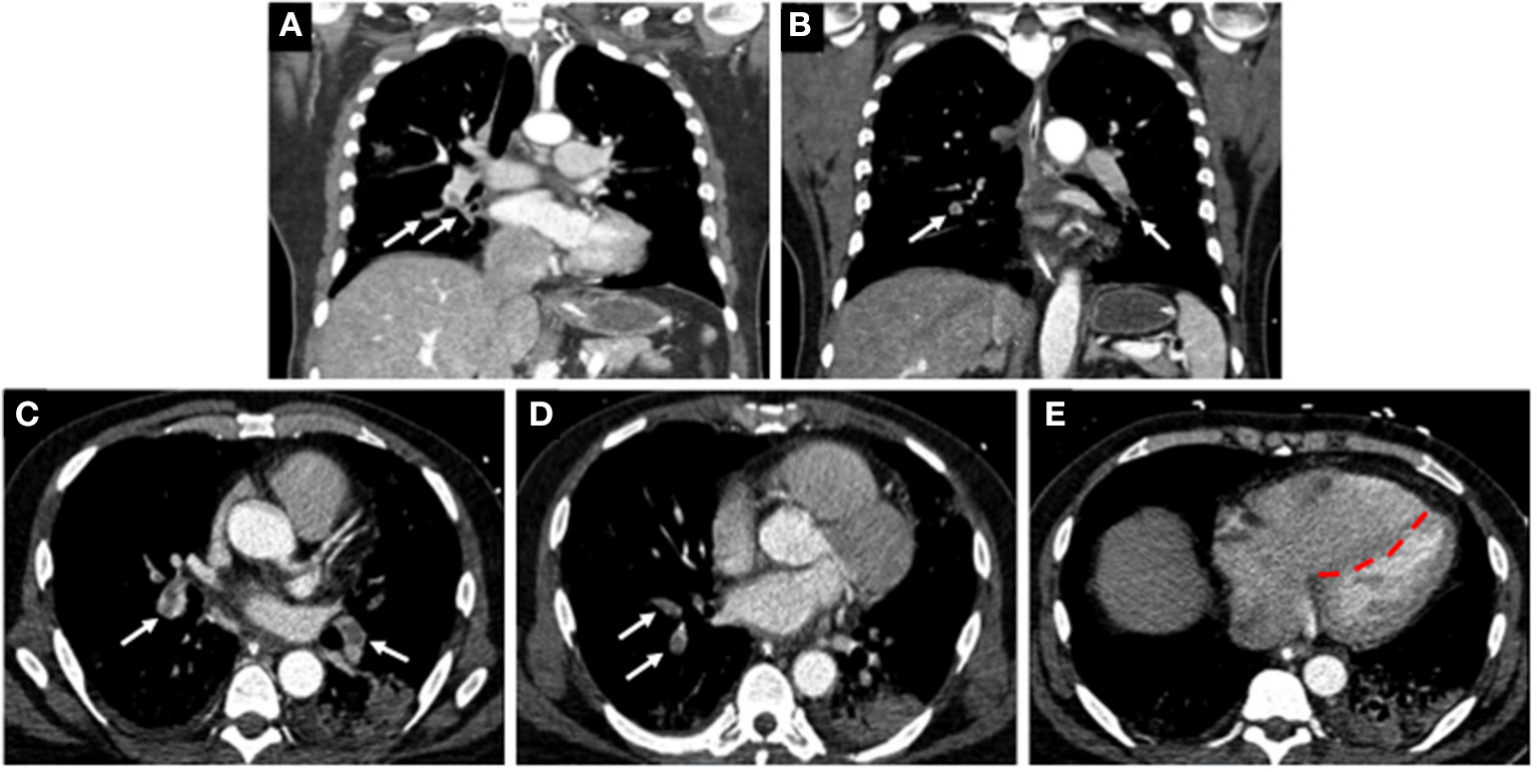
Computed tomographic (CT) images of a COVID-19 positive patient who presented with bilateral lobar pulmonary embolism (white arrows; A–D), and right ventricular enlargement and bowing of the interventricular septum to the left, consistent with right heart strain (red line; E).
